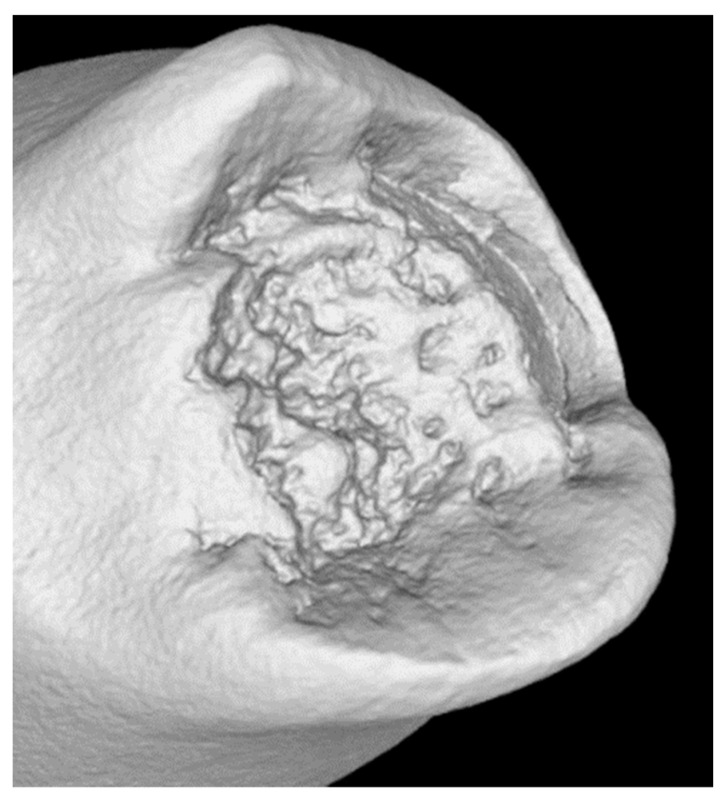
Sample after tensile test CT reconstruction.
